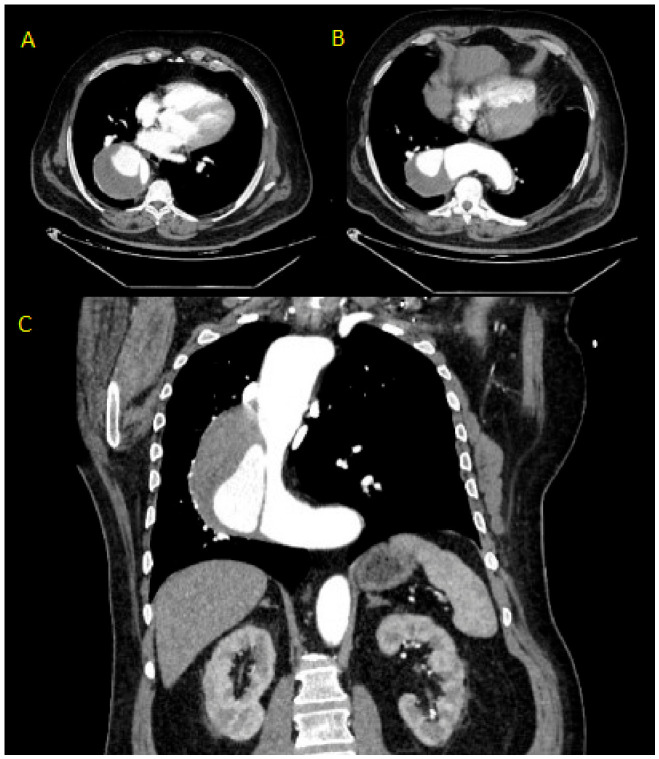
CT angiography ((A): axial; (B): axial at different leve; (C): coronal) demonstrating a saccular aneurysm of the descending thoracic aorta with eccentric mural thrombus and significant luminal compromise. Saccular configuration and thrombus heterogeneity are recognized imaging features associated with focal wall instability and increased rupture risk, supporting the role of CTA in risk stratification and management planning.
